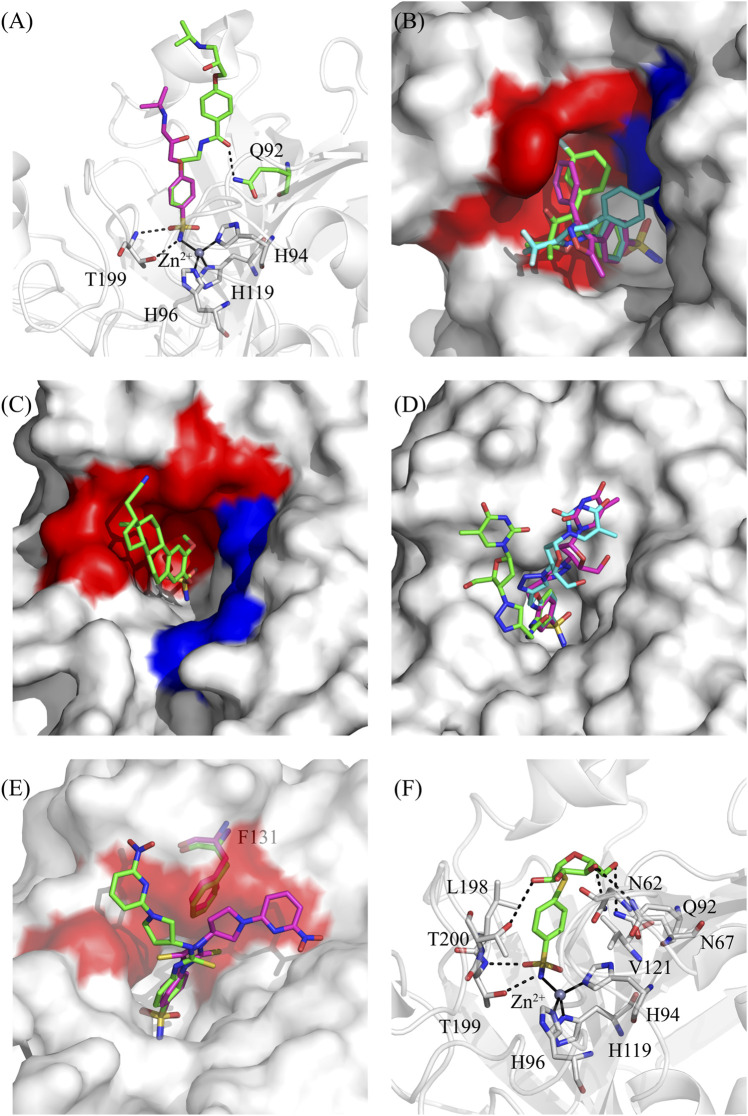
X-Ray structures of representative dual inhibitors bound to hCA II. (A) Superposition between hCA II/1 (green, PDB code 5WLV) and hCA II/2a (magenta, PDB code 5WLT). The hydrogen bond between compound 1 and Gln92 is shown as a dotted black line. Zinc ion coordination and hydrogen bond interactions between Thr199 and sulfonamide moiety are also reported. (B) Superposition of celecoxib (cyan, 1OQ5 PDB code), valdecoxib (magenta, 2AW1 PDB code) and palmacoxib (green, 5GMN PDB code) in complex with hCA II. The protein accessible surface is shown with hydrophobic residues (Ile91, Val121, Phe131, Val135, Leu141, Leu198) coloured in red, and hydrophilic ones (Asn67, Glu69, Gln92) in blue. (C) Accessible surface of hCA II in complex with compound 3b (PDB code 3BET), chosen as representative inhibitor of hCA/STS-dual inhibitors. Residues delimiting the hydrophobic and hydrophilic regions of hCA II active site cavity were coloured in red and blue, respectively. (D) Superposition of hCA II bound to dual inhibitors targeting hTL: hCA II/8a (cyan, PDB code 6YPW), hCA II/8b (magenta, PDB code 6WKA) and hCA II/8c (green, PDB code 7NH6). Accessible surface of hCA II is also depicted. (E) Superposition between hCA II/(*R*)-10a (green, PDB code 8BJX) and hCA II/(*S*)-10b (magenta, PDB code 8BOE). Phe131 of both adducts and the double conformation of thioureido moiety observed for compound (*S*)-10b are shown. Accessible surface of hCA II is also depicted with residues delimiting the hydrophobic region of hCA II active site cavity in red. (F) Active site view of hCA II/12a (PDB code 7ZWB). Residues involved into ligand binding are depicted, with hydrogen bonds shown as dotted black lines. Zinc ion coordination is also reported. Figures were made by using PyMol. SASA was calculated with a default probe radius of 1.4 Å.
